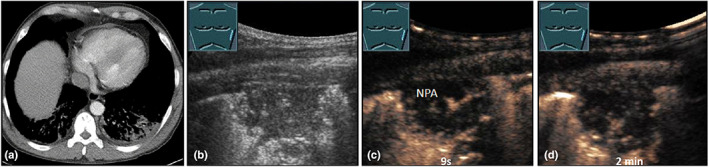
A 29‐year‐old male patient presented to the emergency department with respiratory‐dependent severe thoracic pain with fever, in the setting of known homozygous sickle cell disease. Left‐sided, dorsal, peripheral lung consolidation was seen on computed tomography (courtesy of Prof. Dr. Andreas H. Mahnken, Department of Radiology, University Hospital Marburg) (a); B‐mode ultrasound (b) shows a hypoechoic, irregularly bordered, inhomogeneous consolidation; contrast‐enhanced ultrasound shows only marginal pulmonary arterial perfusion after 9 seconds (c), with a large, central non‐perfused area also visible after 2 minutes (d), consistent with infarct pneumonia caused by sickle cell crisis.
